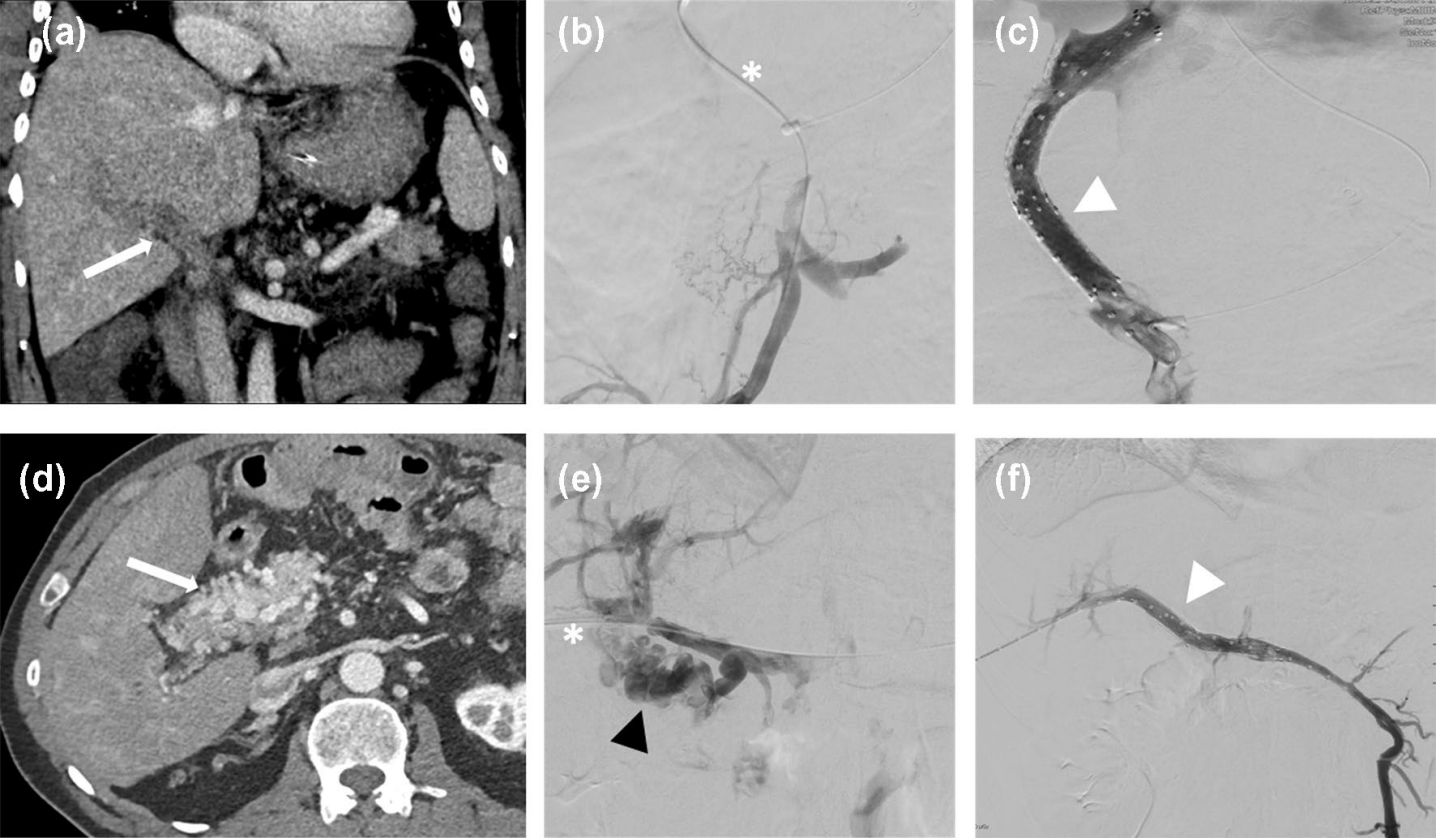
Exemplary cases of two patients with PVT included in this study who were treated with different recanalization techniques that include two interventional access routes (PT- and TIPS access). The first patient aged 50 (patient number 12/a–c) developed acute 2 PVT due to sepsis. a Initial CT with complete thrombotic obstruction of the extra- and intrahepatic portal venous system (white arrow). b Initial angiogram after TIPS access (asterisk) showing obstruction of the portal vein and the subsequent collateralization. c Angiogram showing successful patency of the portal vein achieved after thrombectomy, TIPS-Implantation and stenting up into the superior mesenteric vein (white arrowhead). The second patient aged 60 (patient number 10/d-f) with chronic portal vein thrombosis first diagnosed in 2011 and subsequent cavernous transformation (grade 3). Interventional recanalization was performed due to unsuccessful anticoagulation therapy and escalating portal hypertensive hemorrhagic gastropathy. d CT scan showing complete thrombotic obstruction of the portal vein with subsequent cavernous transformation. e Initial angiogram after percutaneous transhepatic access (asterisk) showing the cavernous transformation of the portal vein (black arrowhead). f Angiogram showing the successful patency of the portal vein achieved after porto-mesenteric stenting (white arrowhead). *CT* computed tomography, *PVT* portal vein thrombosis, *TIPS* transjugular intrahepatic portosystemic shunt
